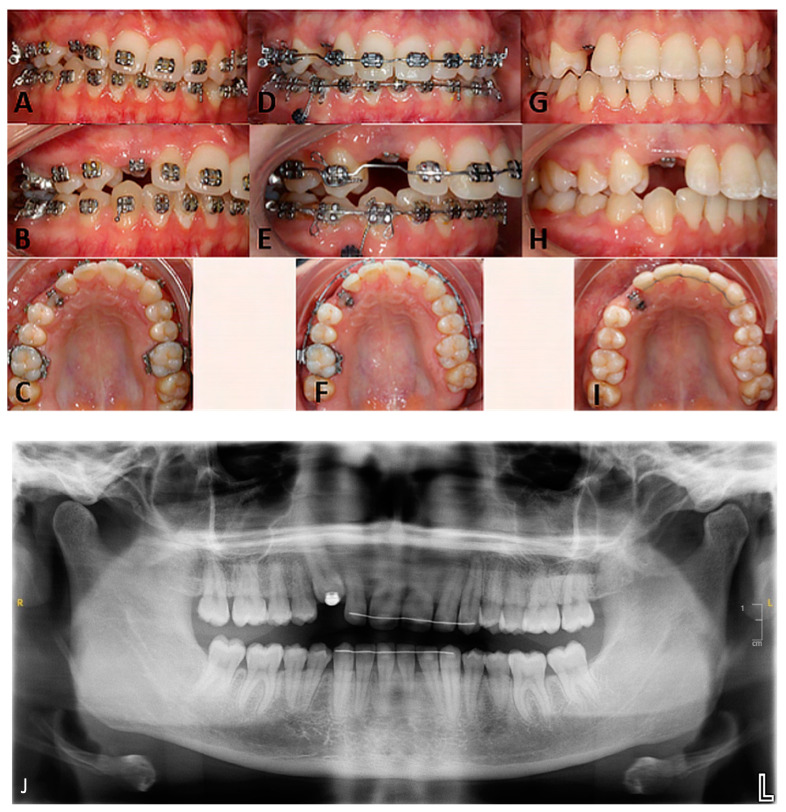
Frontal intraoral views in occlusion, right lateral views, occlusal views of the maxillary arch: (A–C)—initial situation, at the first consultation; (D–F)—intermediate situation, during the second orthodontic treatment; (G–I)—clinical situation upon debonding, (J)—post-treatment orthopantomogram.
